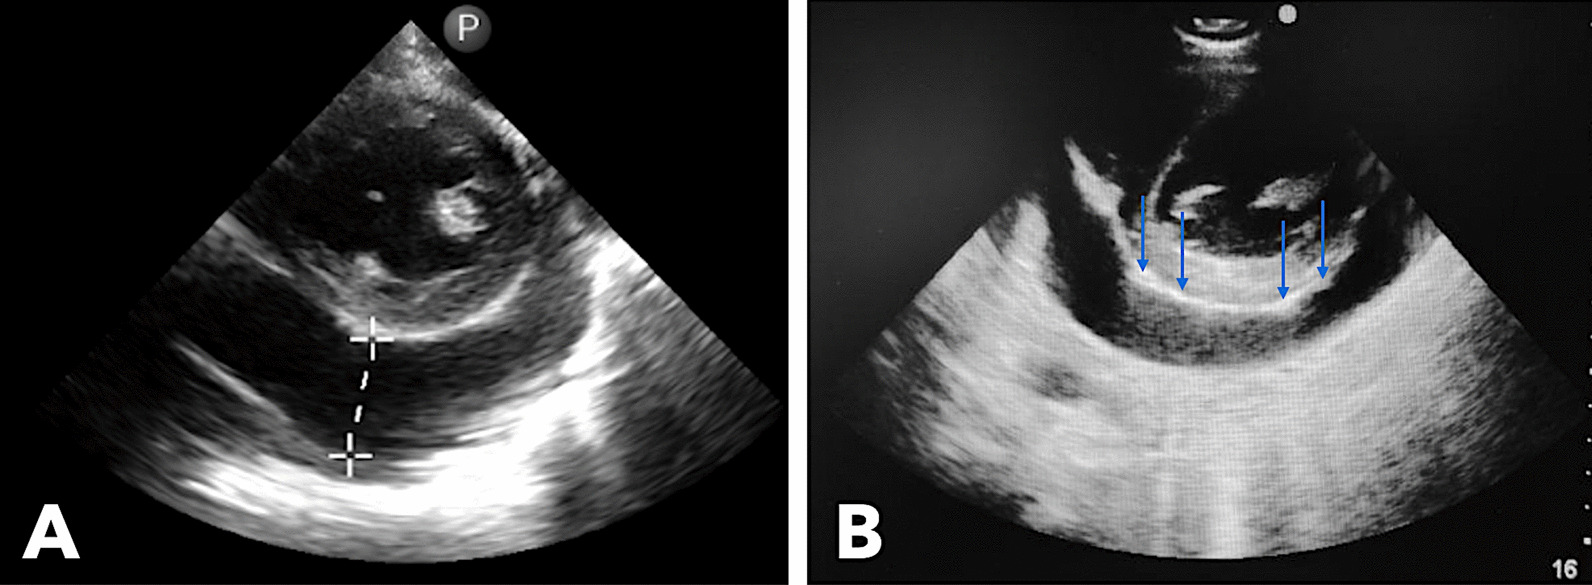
Echocardiography performed showing 3.1-cm echo-free space in the posterior pericardial space (A) and thickened pericardium with frond-like fibrinous projections (blue arrows) extending into the parietal pericardium (B)
